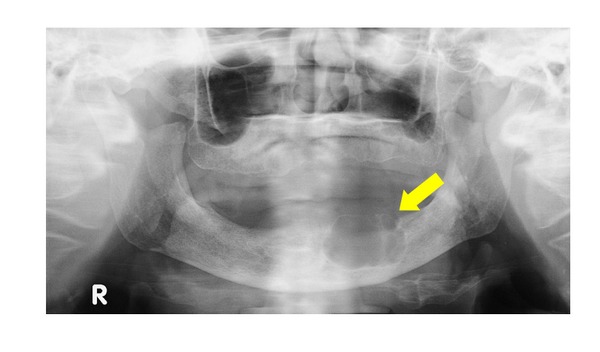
Panoramic X-ray view. A polycystic radiolucent area with an irregular margin can be seen in the molar region of the mandible on the left side.
